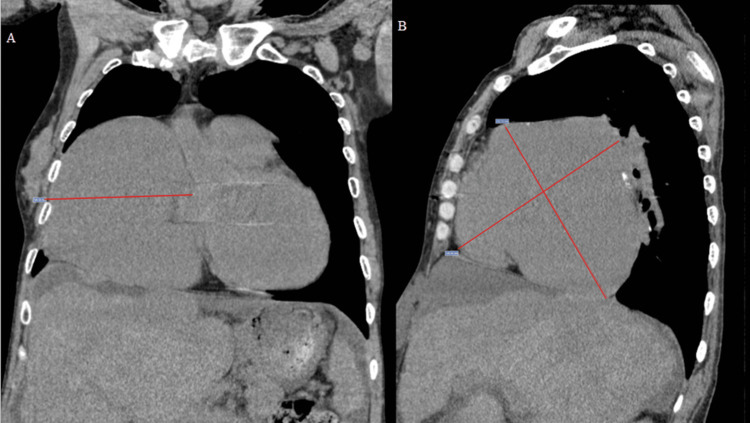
Non-contrast-enhanced chest CT: Coronal (A) and Sagittal (B) views. Estimated right atrium volume was 1,200.7 mL (through ellipsoid volume formula: 0.523 x height x width x length, which was 14.6, 10.4 and 15.2 cm, respectively).
